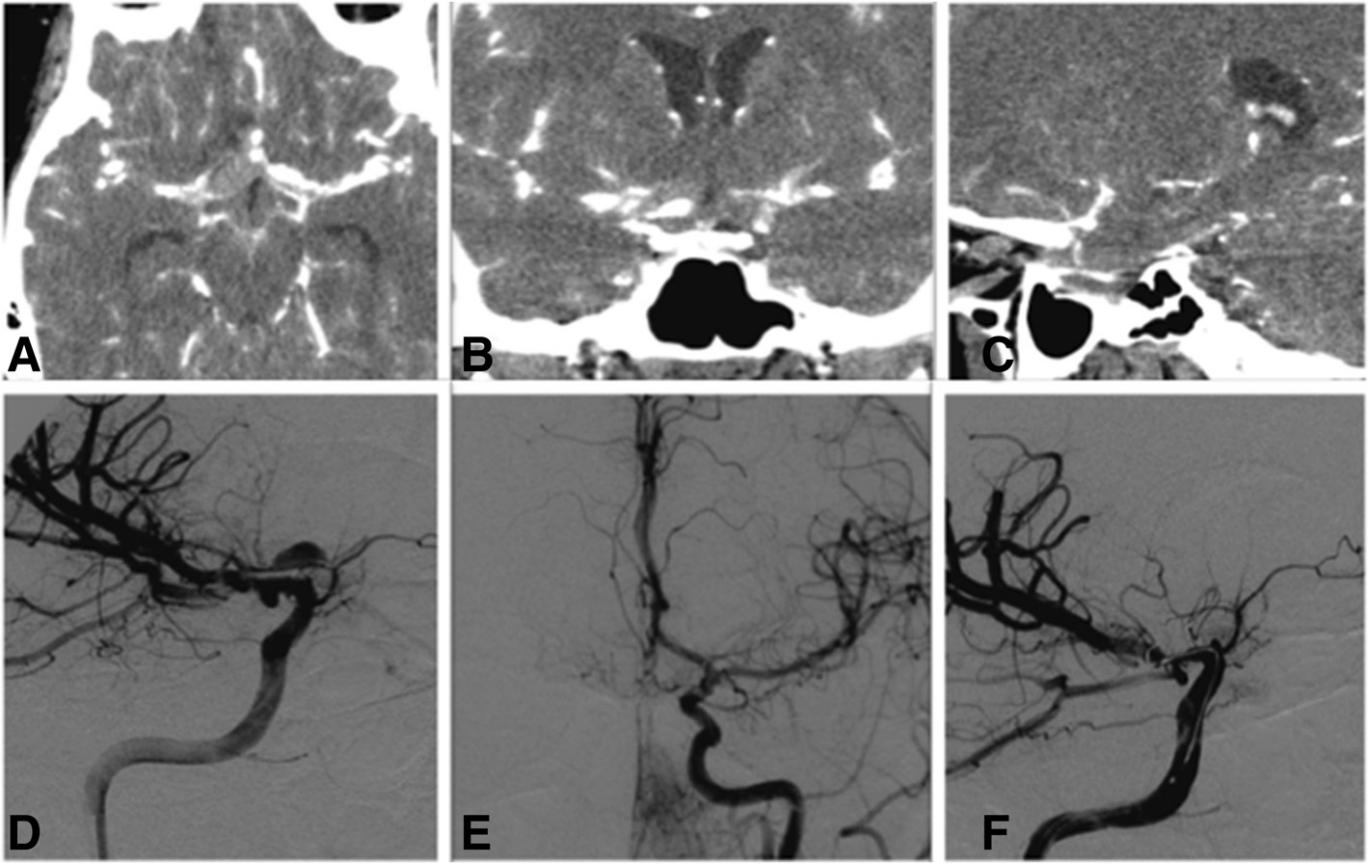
Axial (A), coronal (B) and sagittal (C) computed tomography angiography scans showing a dilated precommunicating segment of the right anterior cerebral artery. An angiogram of the right internal carotid artery (D) showing a false lumen at the level of the precommunicating segment of the right anterior cerebral artery suspected to be a dissecting aneurysm. An angiogram of the left internal carotid artery (E) demonstrating that both right and left postcommunicating segments of the anterior cerebral artery are perfused from the left anterior cerebral artery. An angiogram of the right internal carotid artery (F) showing the impossibility of accessing the aneurysm via an endovascular route due to its characteristic features and the vasospasm.
